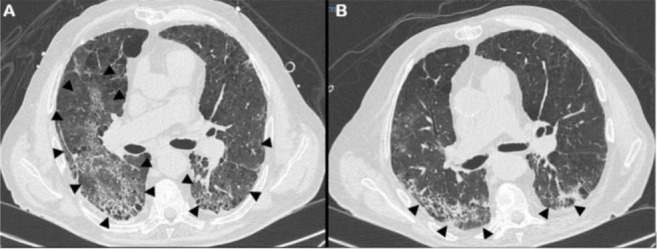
Pulmonary high resolution computed tomography. (A) Bilateral ground glass area affecting the inferior lobes (arrows). (B) Almost complete resolution of the ground glass area, and residual fibrotic phenomena (arrows).
